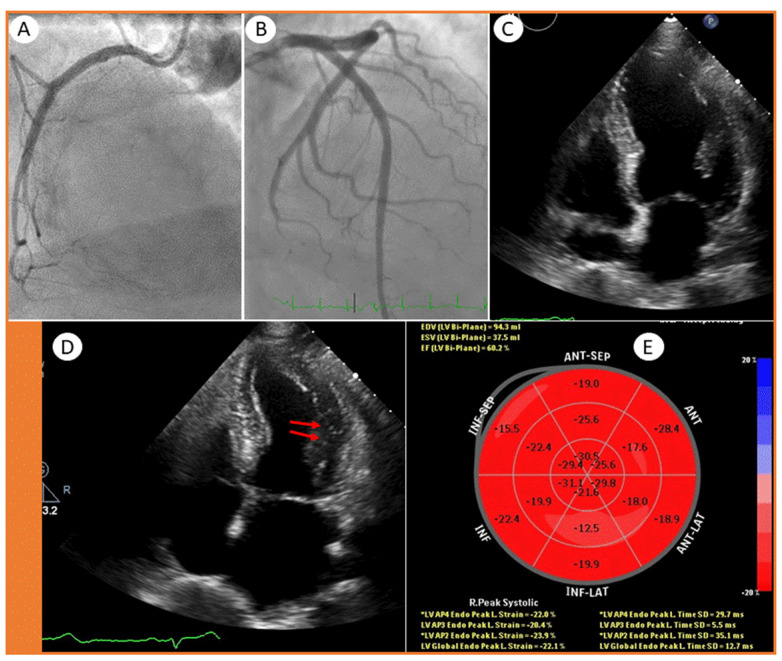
Coronary angiography showing a (A) normal right coronary artery and (B) normal left main stem, left circumflex artery with marginal branches and left anterior descending artery with diagonal branches. Echocardiographic 4-chamber views showing (C) end-diastolic and (D) end-systolic frames with mild hypokinesis (arrows), and a (E) Bulls’ eye plot.
